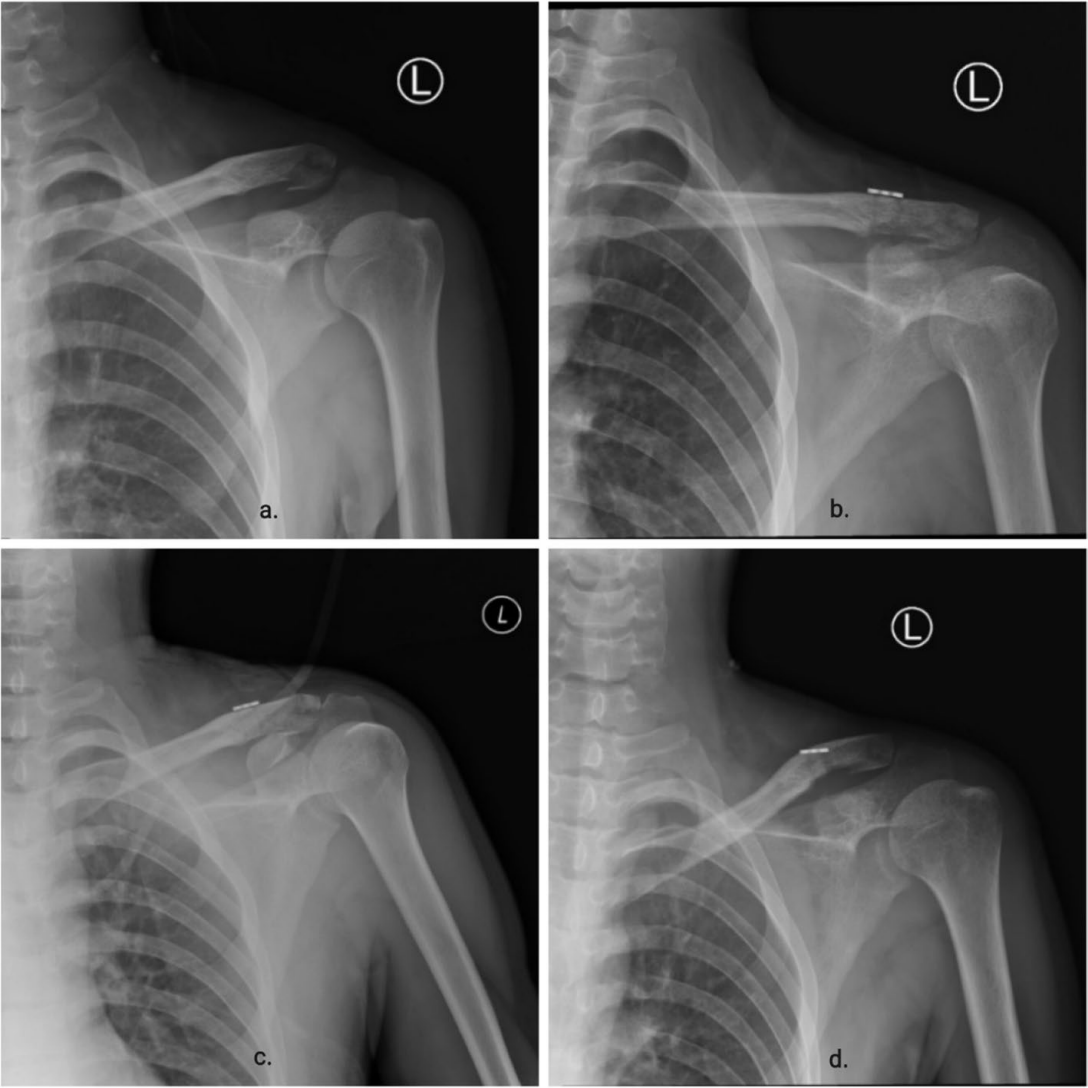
Imaging follow-up of patients. a Pre-operative X-ray showed dislocation of the acromioclavicular joint with distal clavicle fracture. b X-rays on the second postoperative day showed a good dislocation of the acromioclavicular joint and a fracture of the distal clavicle. c X-ray 40 days after surgery shows bone scab formation and fracture lines are blurred. d Postoperative x-ray at 3 months showed almost healing of the distal clavicle fracture and good repositioning of the acromioclavicular joint
